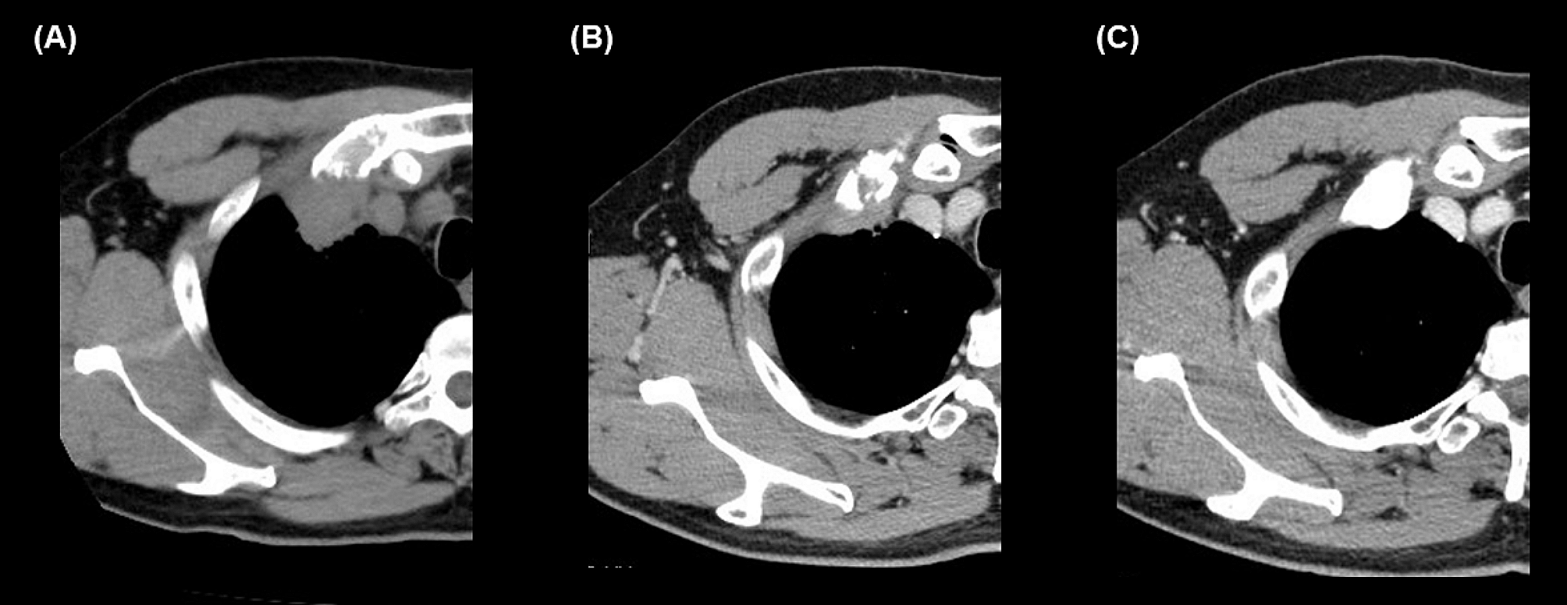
Clinical course. Computed tomography on the 12th day post-admission shows a tumoral lesion suspicious of infiltration into the right first rib and intercostal muscles (A). On the 47th day post-admission, computed tomography shows that the mass had shrunk and flattened since the previous 8 F-Fluorodeoxyglucose positron emission tomography-computed tomography (B). On the 176th day post-admission, computed tomography shows that the right lung S1 segment has a scar-like appearance (C)
